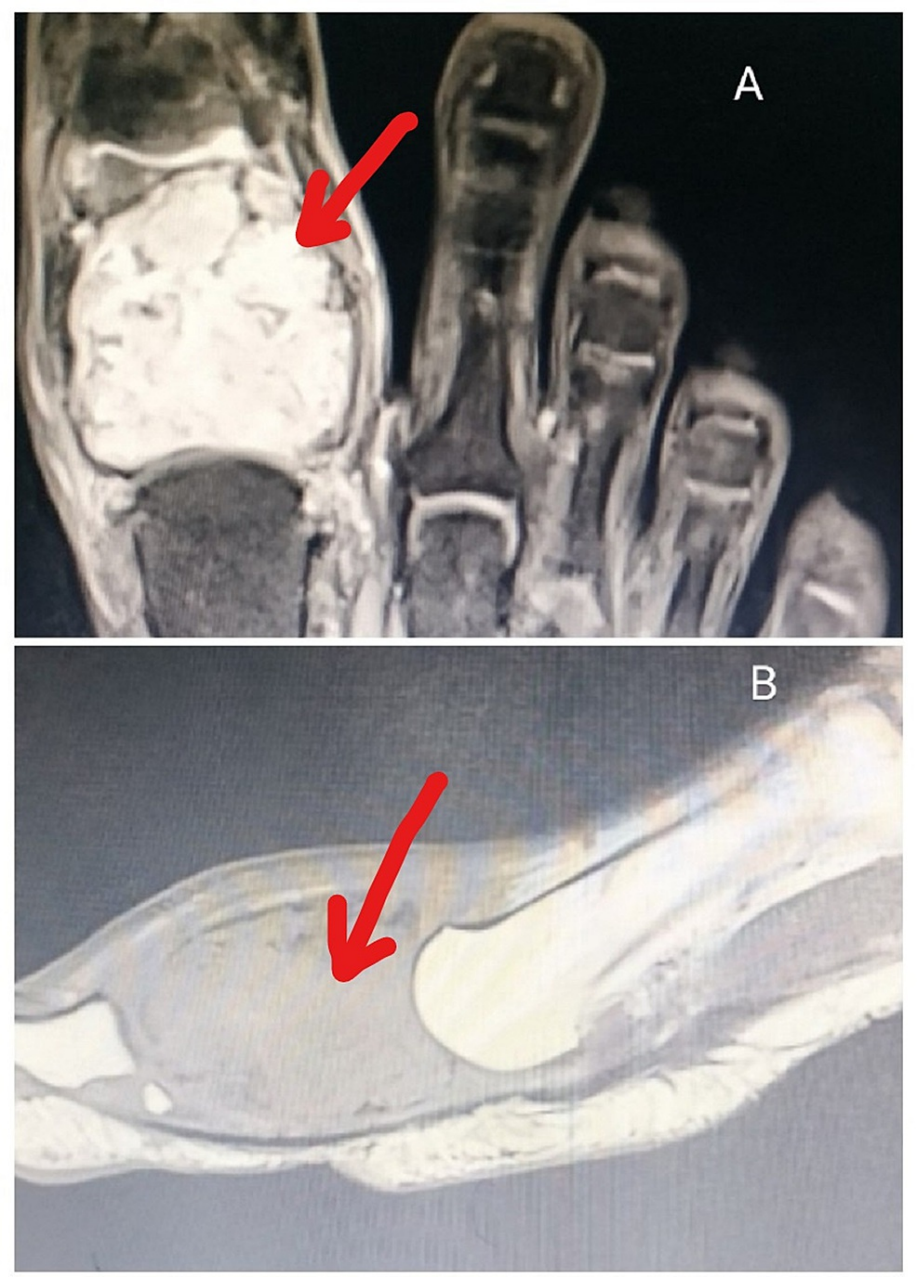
(A) MRI suggestive of a mass is hyperintense on FS-PD, with lesions involving the proximal and distal ends of the phalanx stippled calcification. (B) MRI suggestive of the expansion of the proximal phalanx of the great toe, hypointense on T1, with soft tissue edema and swelling around the proximal phalanx. FS-PD, fat-suppressed proton density; MRI, magnetic resonance imaging
